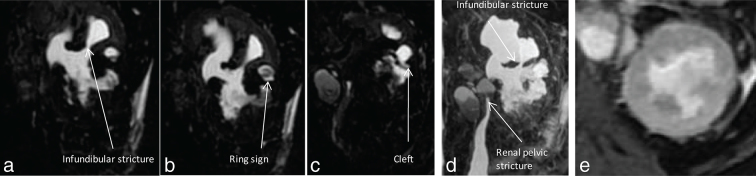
(a–c) showing coronal slices of a 3D magnetic resonance cholangiopancreatography sequence and (d) showing the maximum intensity projection of the 3D magnetic resonance cholangiopancreatography sequence of the left kidney. Seen in (a) is the infundibular stenosis with the triangular papillary cavity corresponding to the CT urogram in Figure 2a. (b) shows a ring sign corresponding to the CT urogram image in Figure 3b with distorted and irregular lower pole calyces. (c) shows a cleft in the interpolar calyx. Also seen in this image is the renal hilar lymph node. (d) shows the renal pelvic and interpolar infundibular stricture with disproportionately dilated upper and lower pole calyces, with irregular contour of the lower pole calyces. [Fig f4] (e) shows *T*_2_ hyperintensity of the renal parenchyma.
